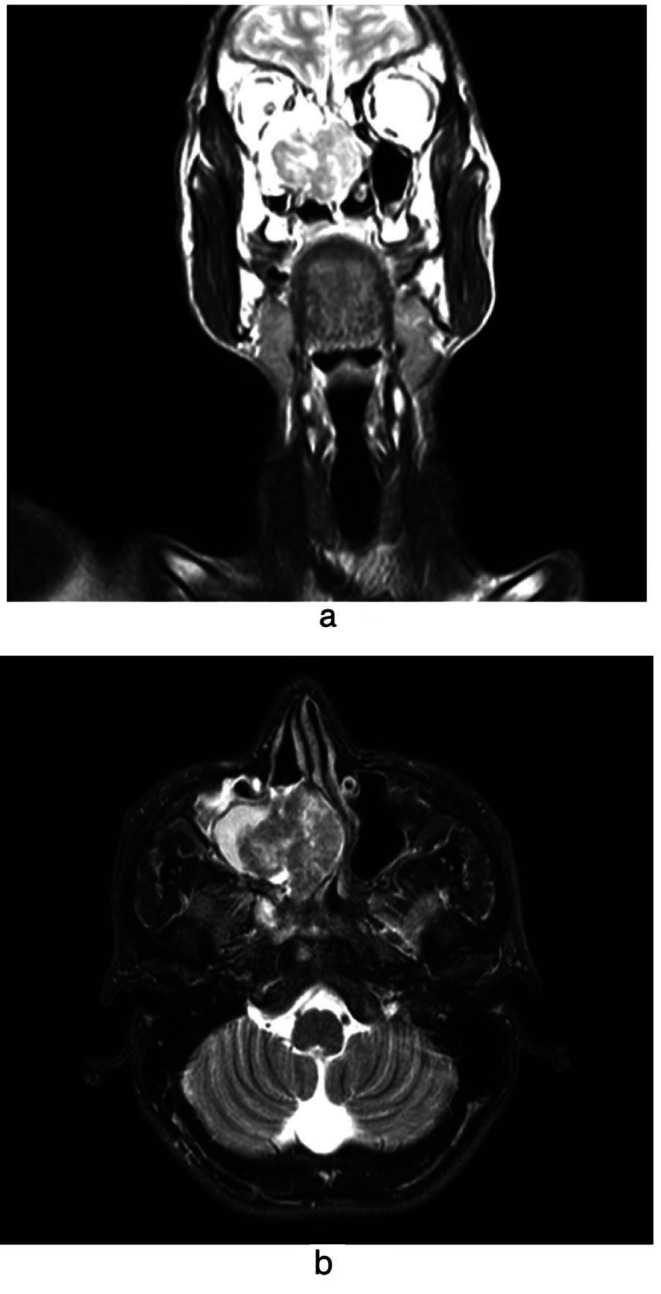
1a: MRI showing lesion in coronal section. 1b: MRI showing lesion in axial section
